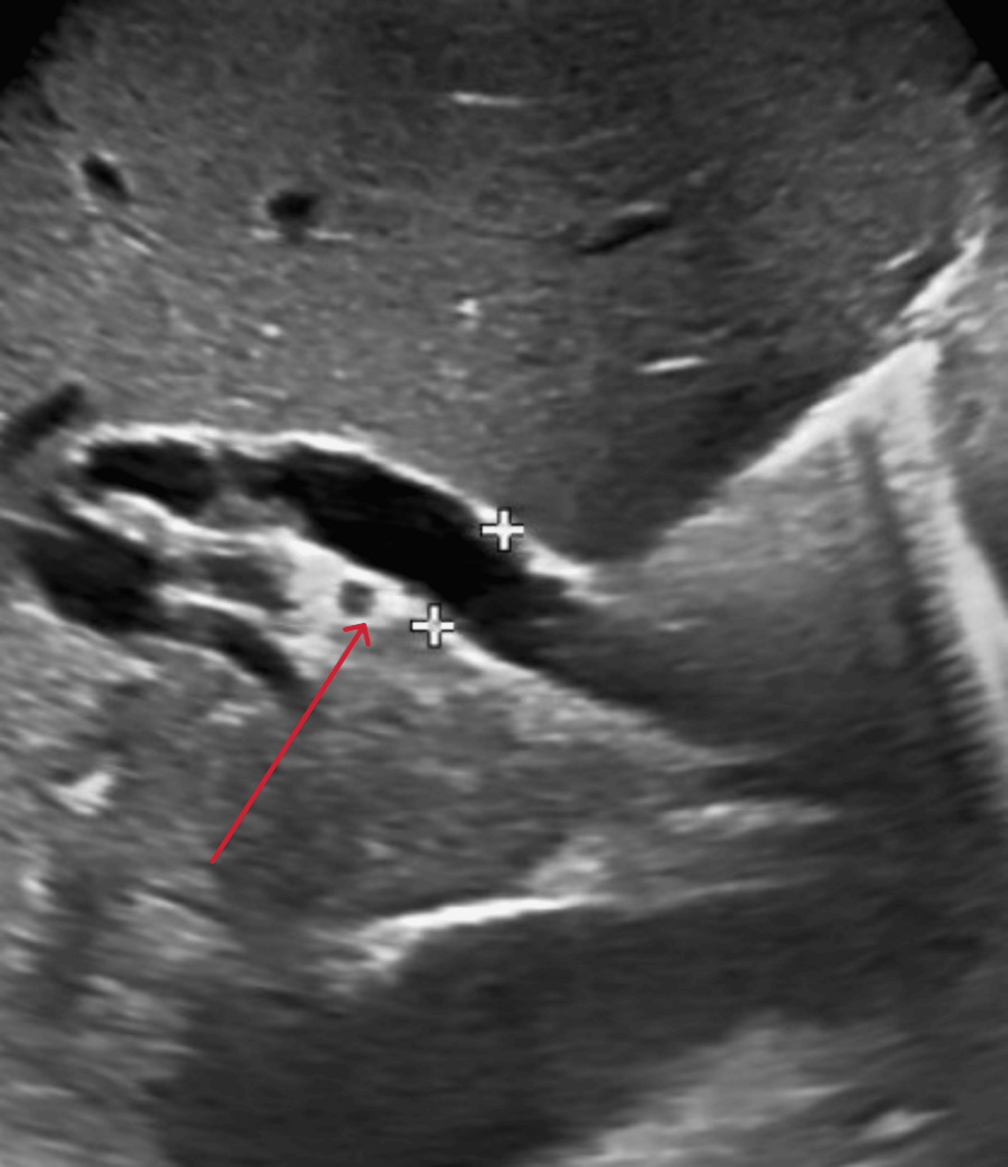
Abdominal ultrasound showing dilated common bile duct Abdominal ultrasound image showing a dilated common bile duct (red arrow), suggestive of possible biliary obstruction or underlying pathology requiring further evaluation.
